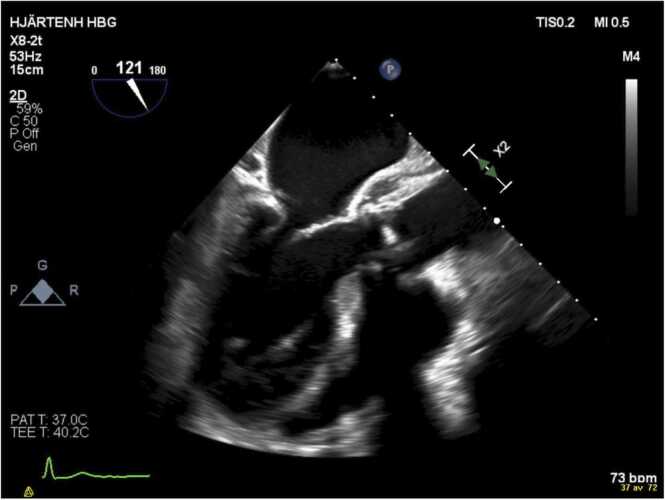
Transesophageal echocardiography of the patient on day 10 of hospitalization.
